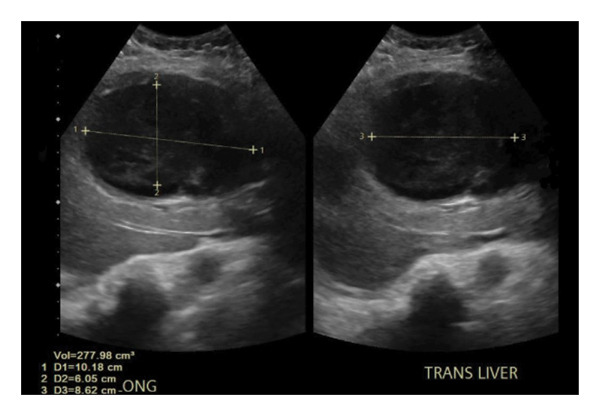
Ultrasound abdomen images demonstrating a markedly distended gallbladder with a thickened wall, heterogeneous internal echoes, and a pericholecystic collection.
